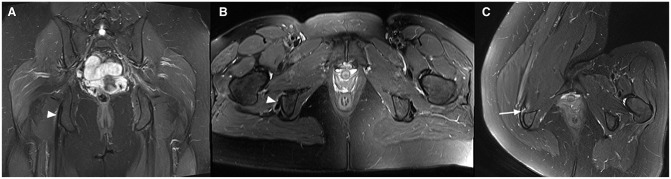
T2-weighted Dixon magnetic resonance imaging scans from a 38-year-old woman with symptoms on the right side. Standard (A) coronal and (B) axial images show mild edema around the proximal hamstring tendons (arrowheads), but the tendons appear quite normal. (C) An axial image with right hip flexion reveals a partial rupture of the semimembranosus tendon (arrow).
